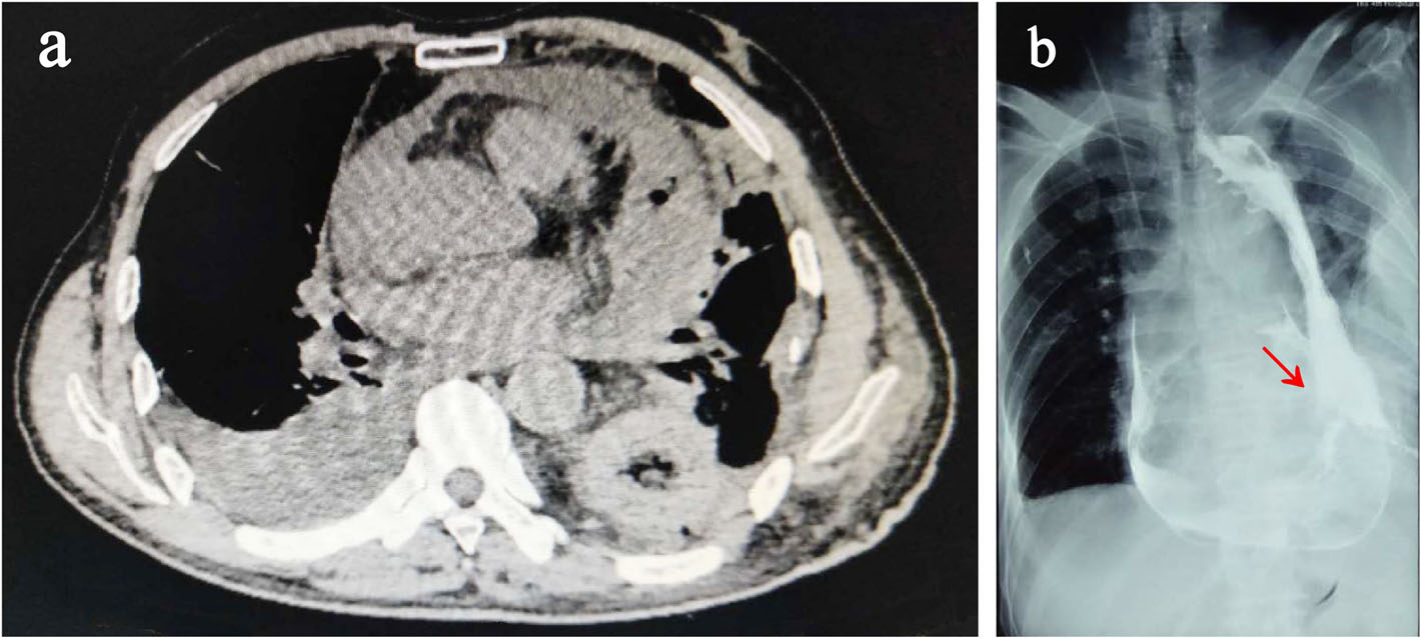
CT and contrast radiography revealed a thoracic gastropericardial fistula. a CT showed moderate pericardial effusion and right pleural effusion. b Contrast roentgenogram showed a thoracic gastropericardial fistula
